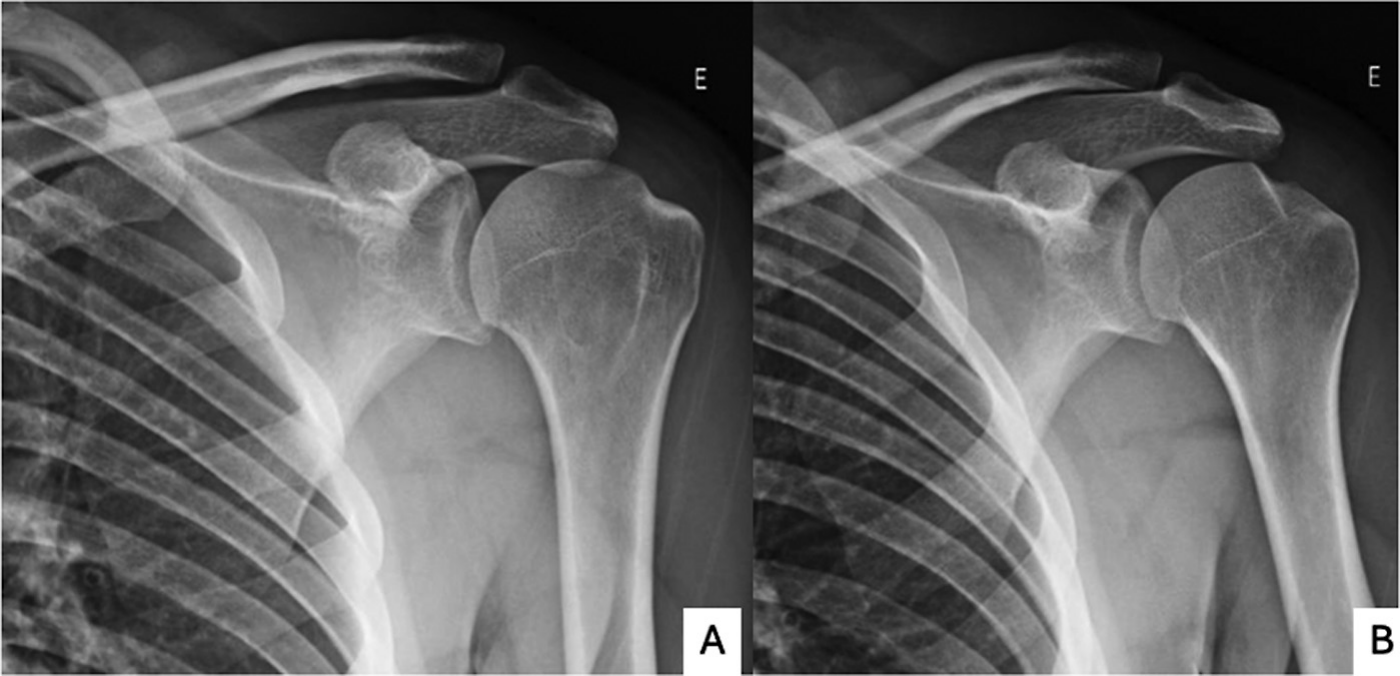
Radiographs of the shoulder with a Hill-Sachs (HS) lesion in true anteroposterior (AP), AP, axillary lateral, and scapular lateral views.
